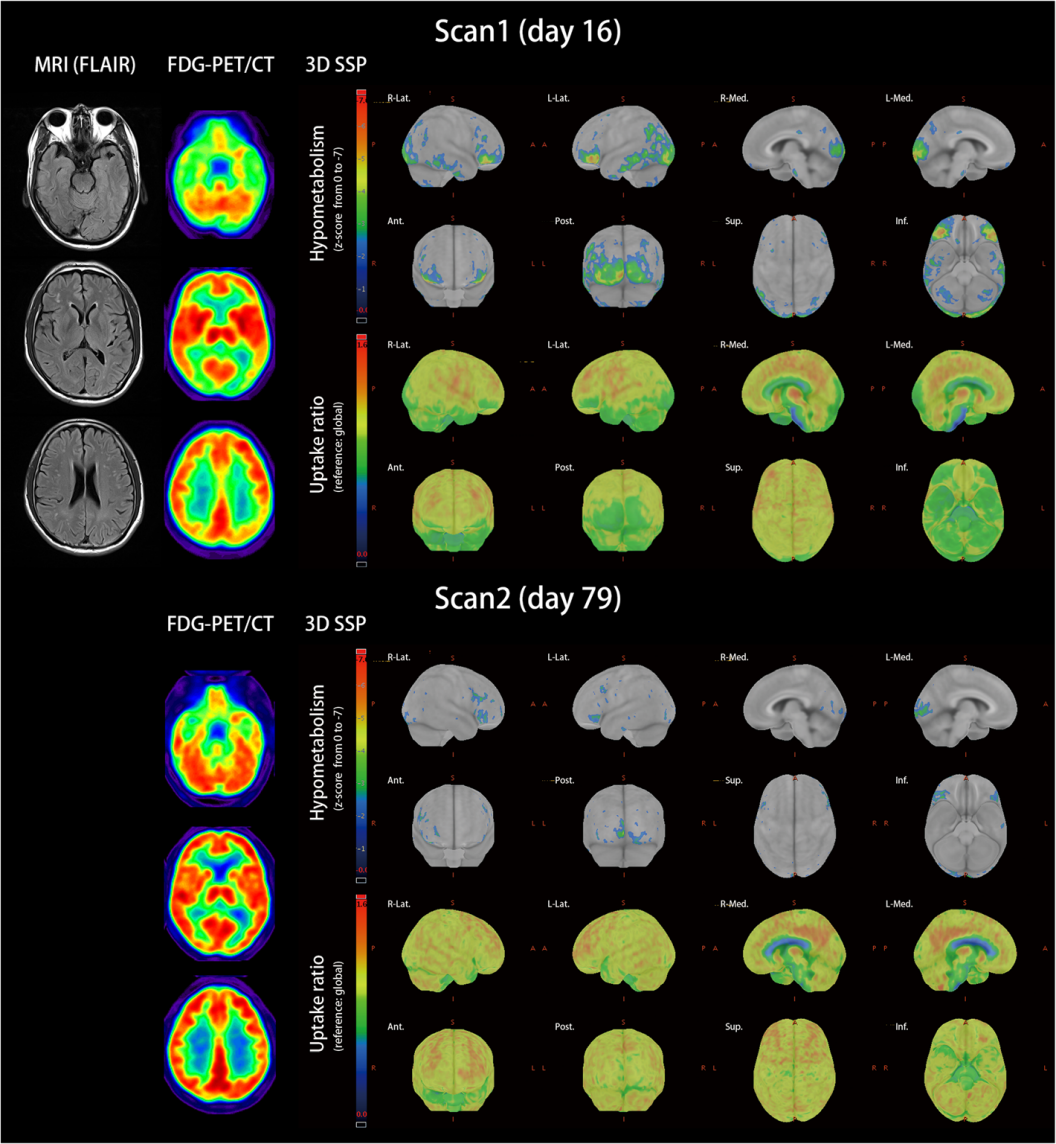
Brain image of MRI and FDG-PET/CT. Initial brain MRI was nonspecific. Fluid-attenuated inversion recovery (FLAIR) imaging did not reveal hyperintensity. FDG-PET/CT was processed through stereo and quantitative analysis by using the standardized z-score with the reference of global brain metabolism. Glucose metabolism was visualized using three-dimensional stereotactic surface projection (3D-SSP). In Scan1 on day 16, the brain exhibited hypometabolism in the medial frontal, orbitofrontal, and occipital lobes and hypermetabolism in the anterior cingulate gyrus and mesial temporal areas. In the first scan, the patient was confused and disoriented. The follow-up scan on day 79 showed resolution of focal hypo- and hypermetabolism. During the second scan, the patient was clear in consciousness, with remaining scanning speech, mild ataxic gait, and some difficulties in calculation
